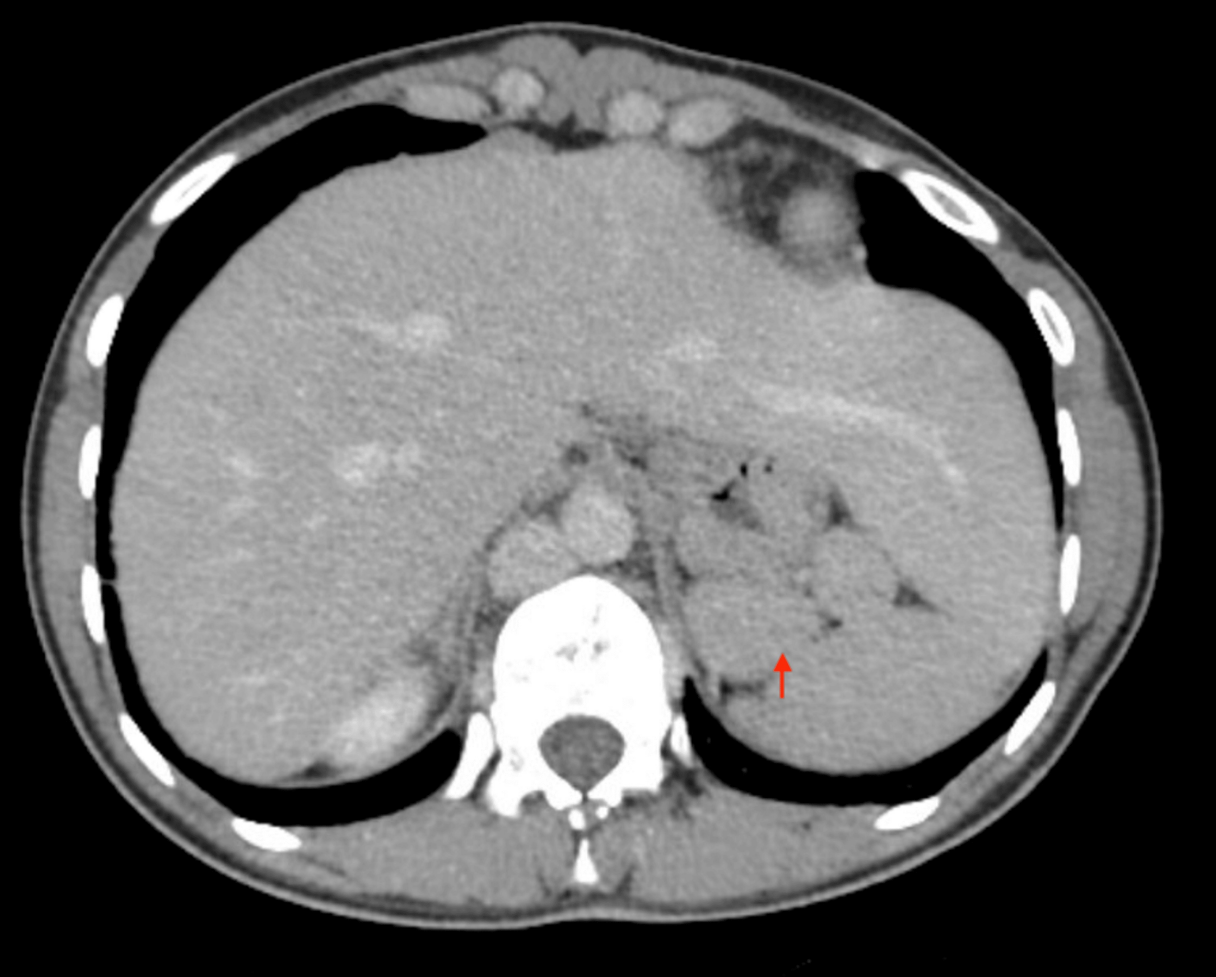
Abdominal CT scan demonstrating the presence of multiple spleens (red arrow) and a liver extending far to the left side.
